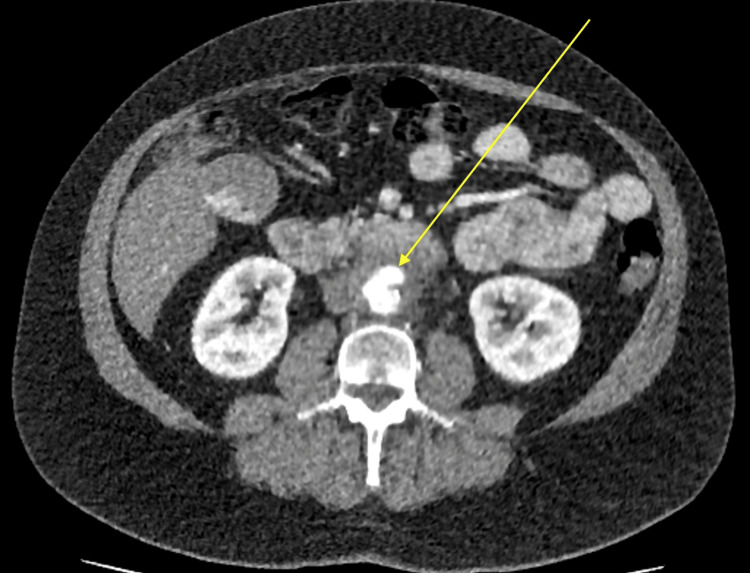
Axial contrast-enhanced CT of the abdomen and pelvis showing increased periaortic fat stranding and asymmetric anterior wall dilatation of the mid-abdominal aorta, concerning for a developing pseudoaneurysm and impending rupture.
